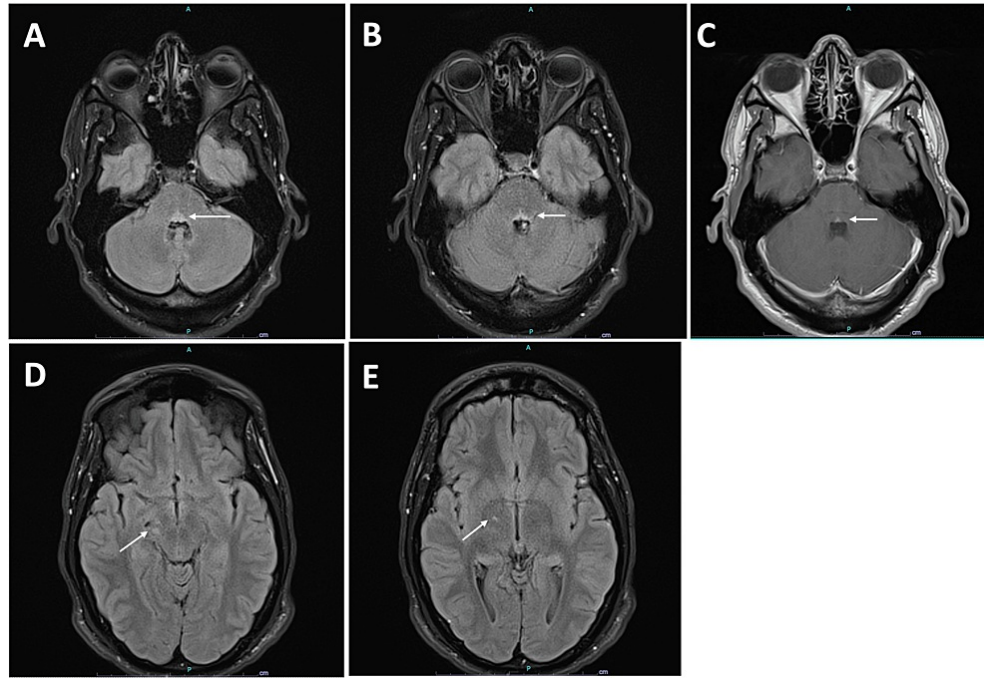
Brain MRI at initial presentation A, B: hyperintensity in the pontine tegmentum along the dorsal pons in the fluid attenuated inversion recovery (FLAIR) sequence. C: subtle contrast enhancement in the dorsal pons in the post-contrast T1 sequence. D: mild hyperintensity in the right cerebral peduncle in the FLAIR sequence. E: a small focus of hyperintensity in the right thalamo-capsular junction in the FLAIR sequence.
